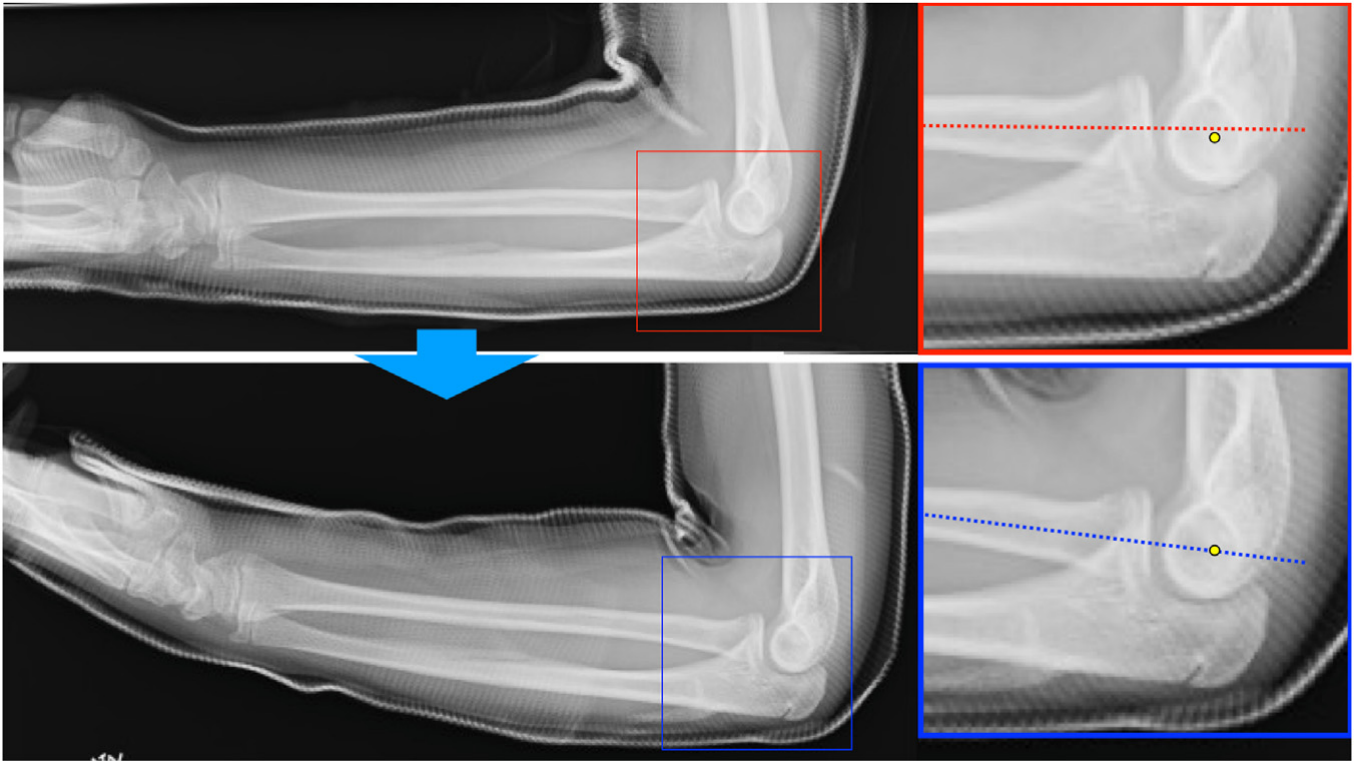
Radiographs were taken on the following day. A slight anterior displacement of the radial head was corrected with the elbow at 100° of flexion and the forearm fully supinated.
